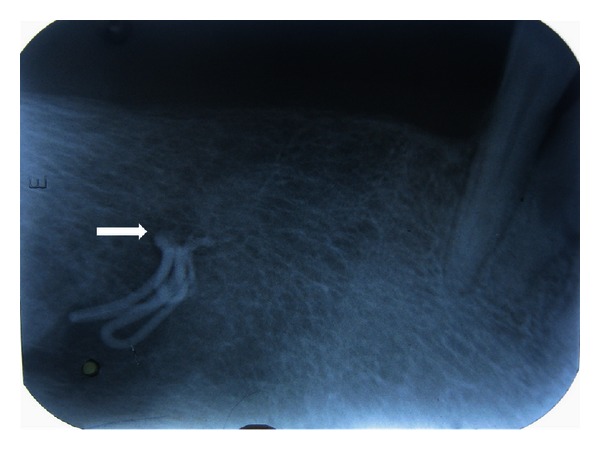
Intraoral radiograph showing the radiopaque foreign body close to the mental foramen.
